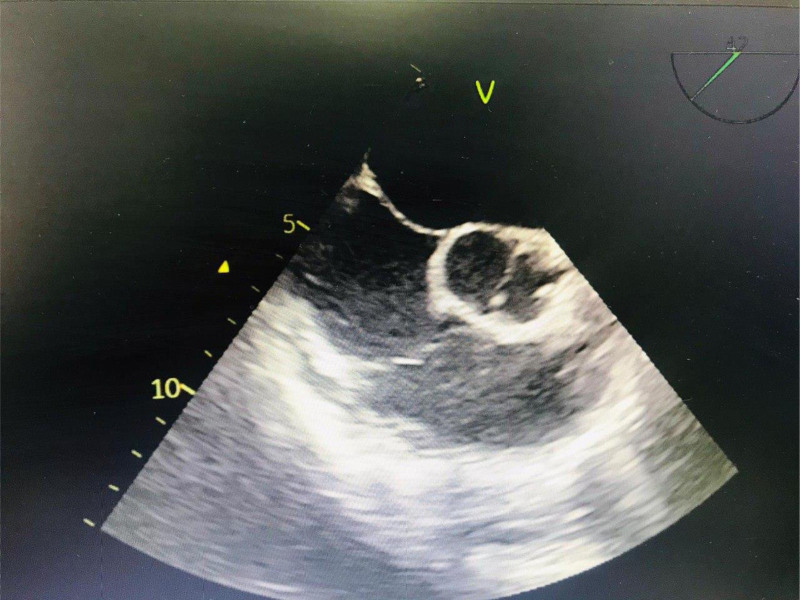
Transthoracic echocardiography showing bicuspid aortic valve.
